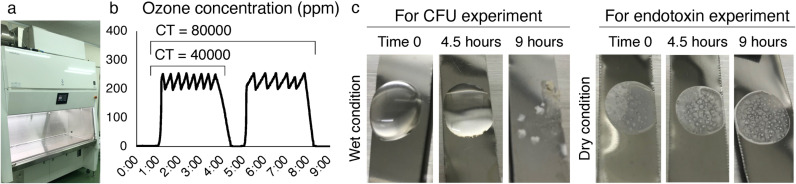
Experimental setup. (A) Medio3 biosafety cabinet equipped with an ozone generator. (B) Ozone generation trends over time, where the x-axis represents time (h), and the y-axis represents ozone concentration in parts per million (ppm). CT denotes concentration-time, calculated as the product of ozone concentration and exposure duration. (C) Experiments for colony-forming units (CFU) and endotoxins conducted under wet and dry conditions, respectively.
